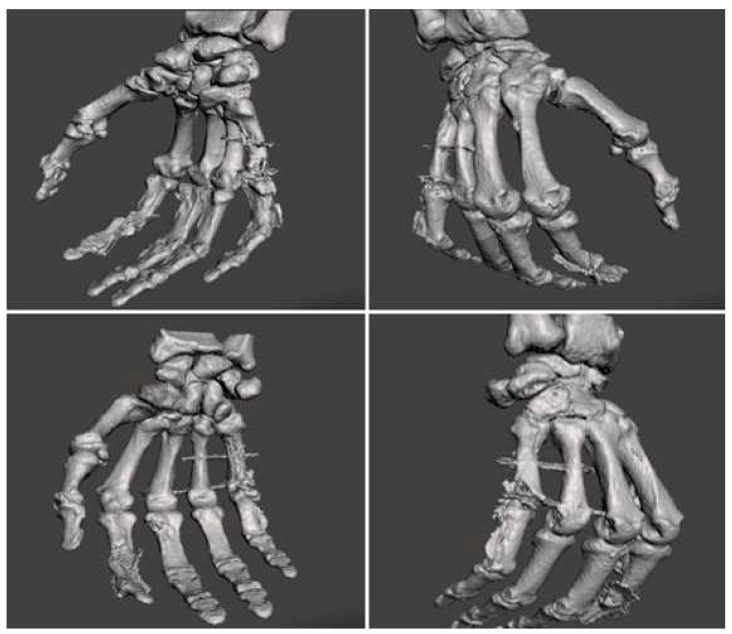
A 3D conversion of the CT images, created for preoperative planning.
